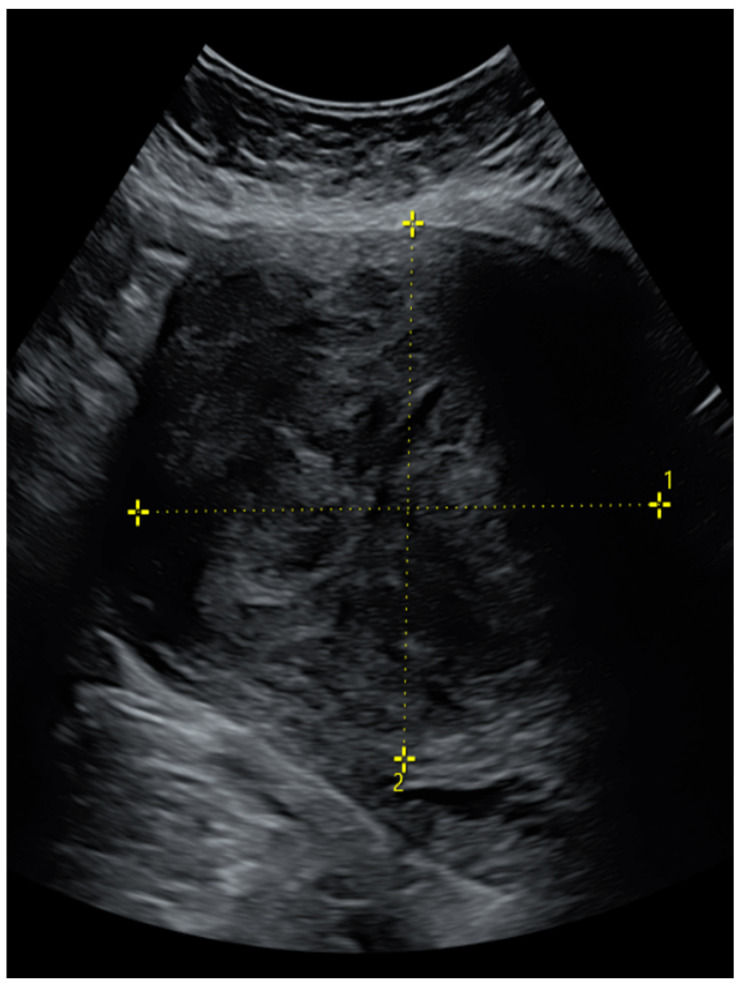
Uterine tumor by trans-abdominal sonography, size approximately 10.89 × 8.96 cm (Longitudinal view, 1: width, 2: height).
